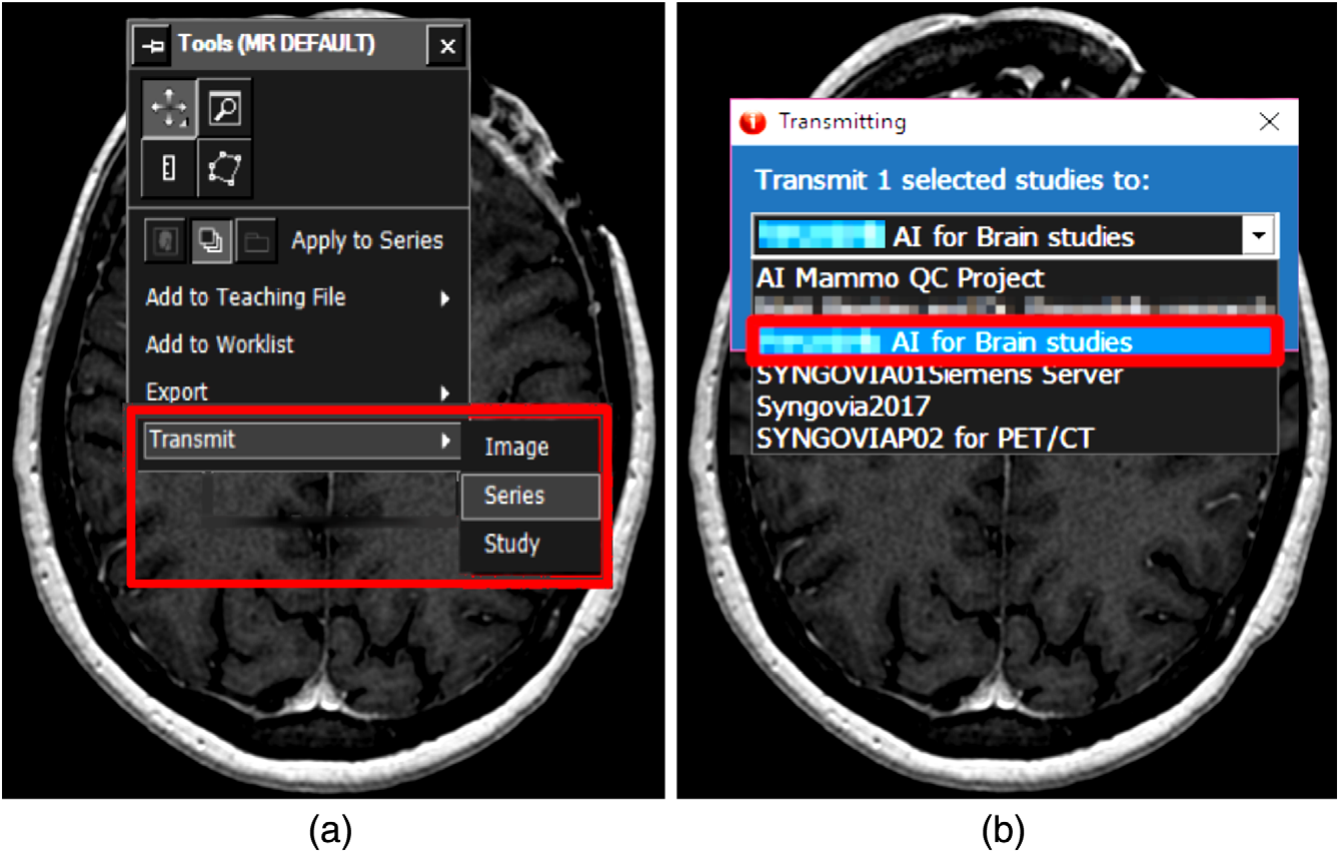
DICOM-transfer to the AI system. (a) DICOM-transfer can be utilized to send the MRI series. (b) The final destination should be the DICOM node where the AI system is located.
